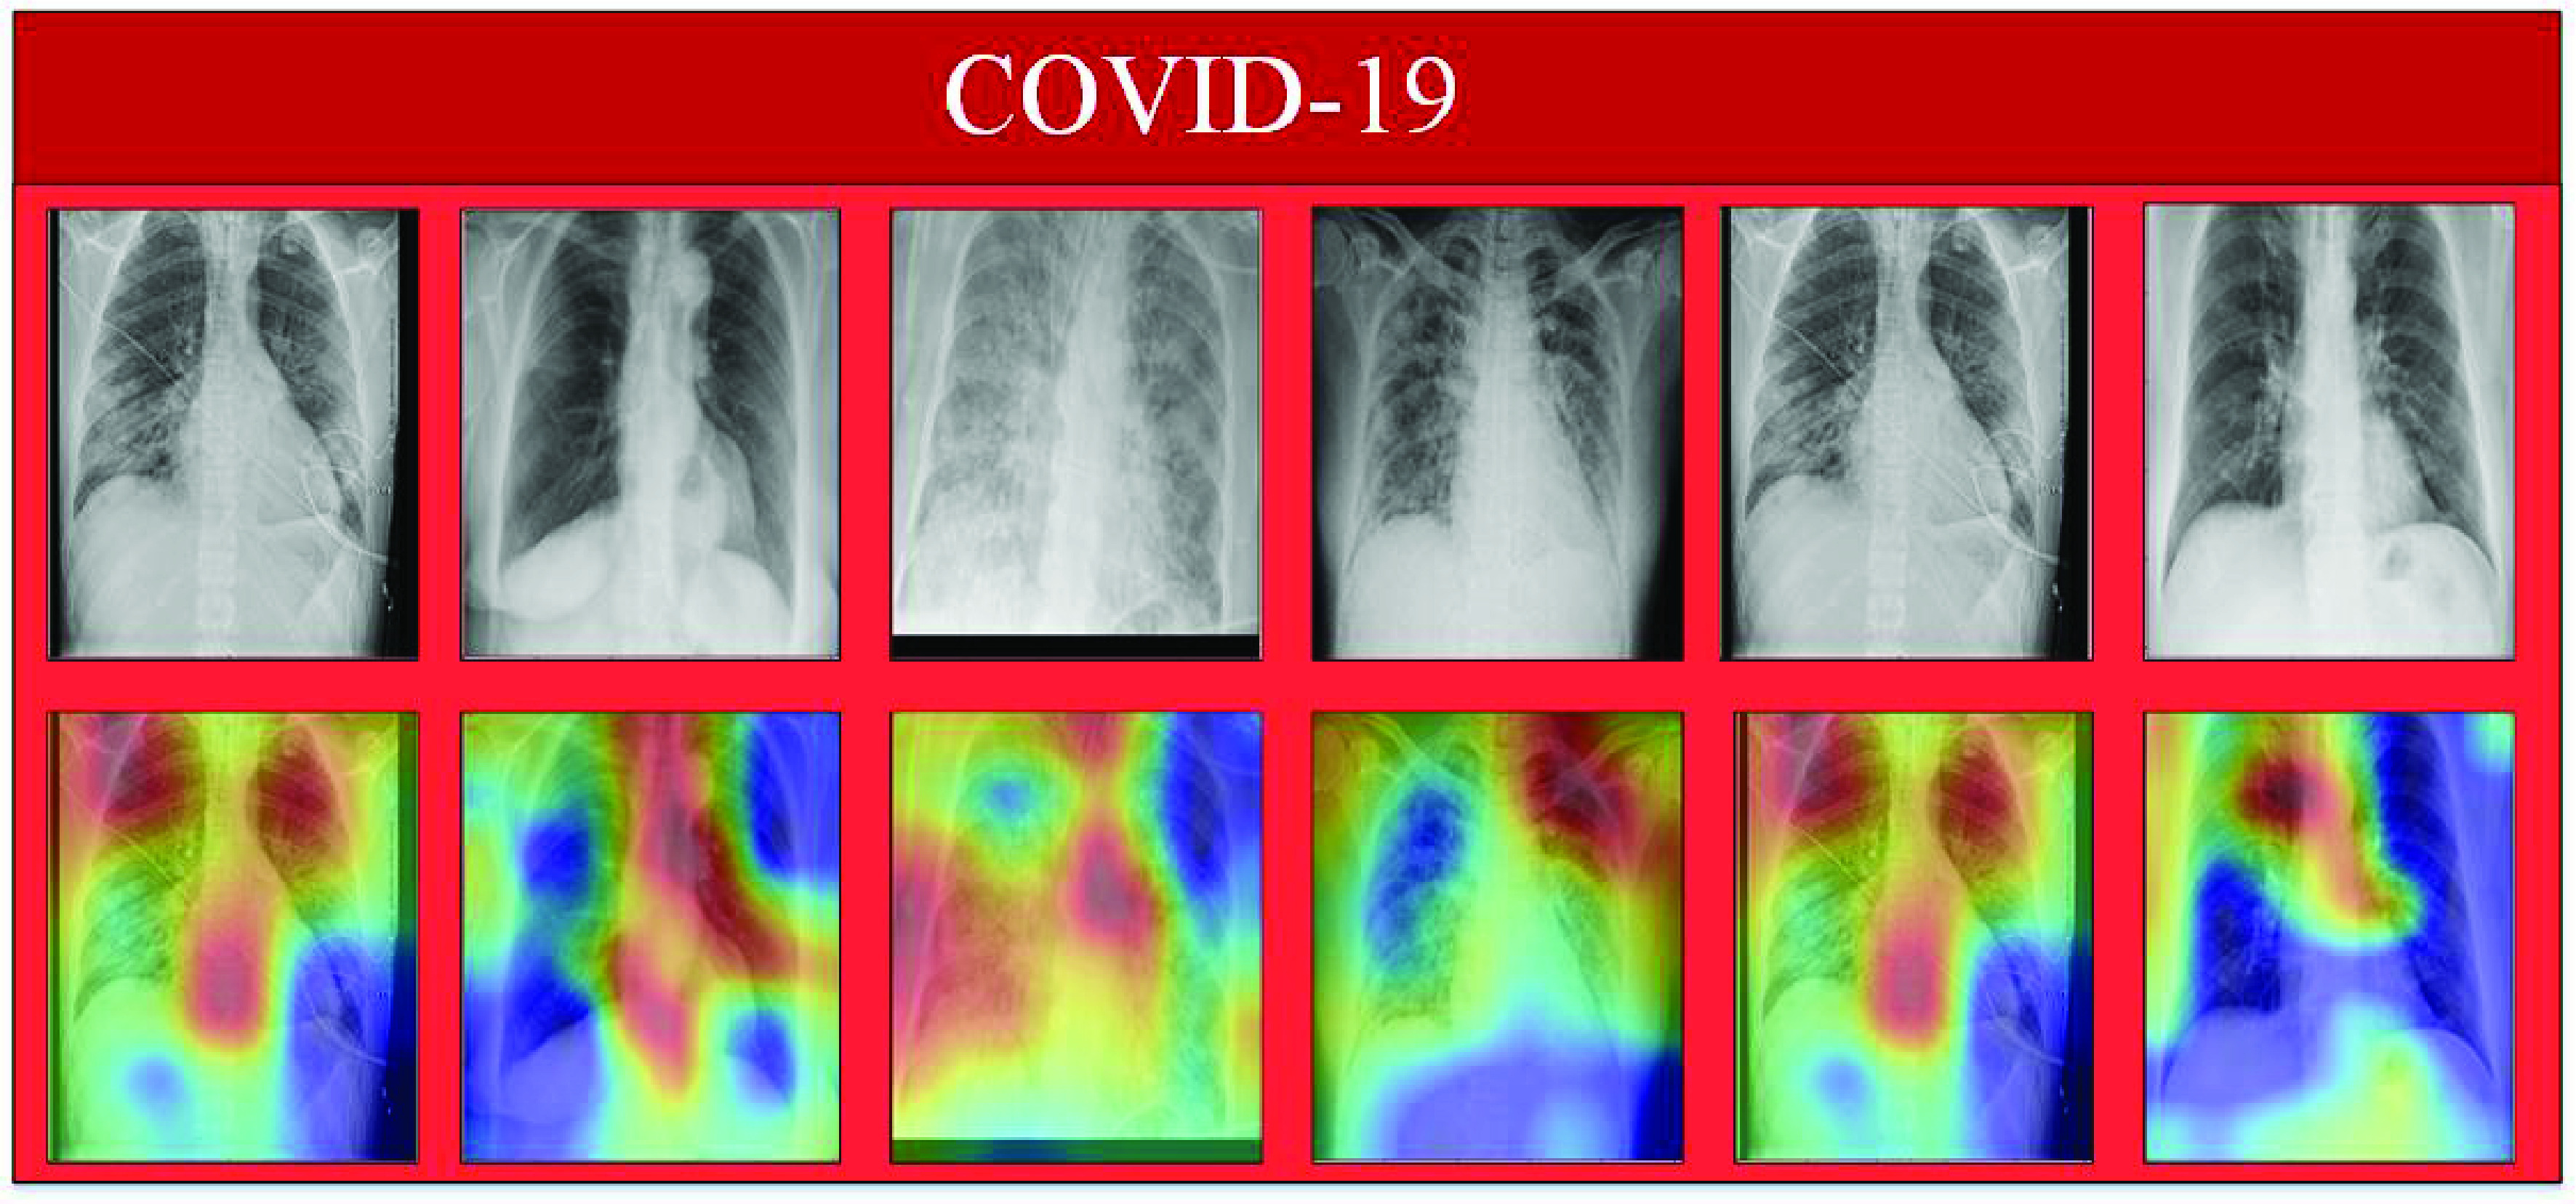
COVID-19 True Positives: correctly classified COVID-19 X-ray scans with corresponding attention maps (CAM). The figure is best viewed in color.
